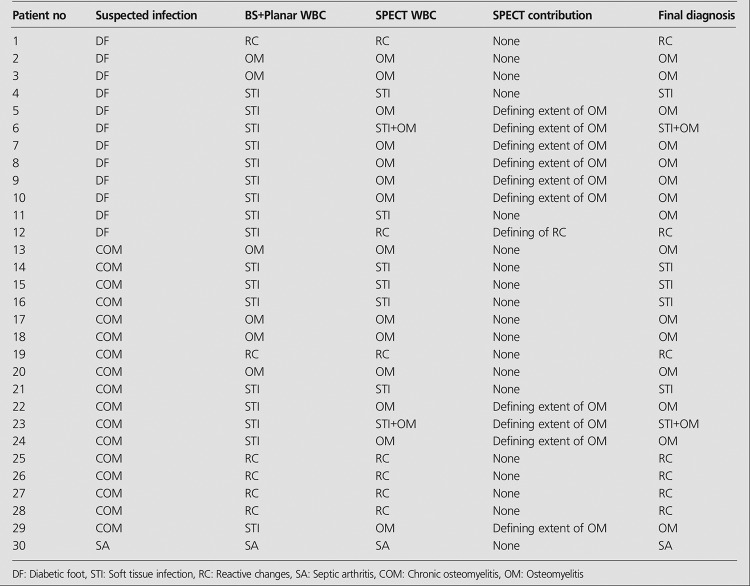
Tc 99m MDP bone scintigraphy of 30-y-old man in whom chronic osteomyelitis in left tibia was suspected. (a) Angiography, (b) blood pool, (c) late phase bone scintigraphy showed increased uptake in left tibia. (d) Tc 99m planar WBC scan showed increased uptake mainly in the soft tissue of left medial cruris. (e) SPECT WBC image localized abnormal uptake in soft tissue and left tibial region. Final diagnosis was found to be soft tissue infection and steomyelitis by biopsy
